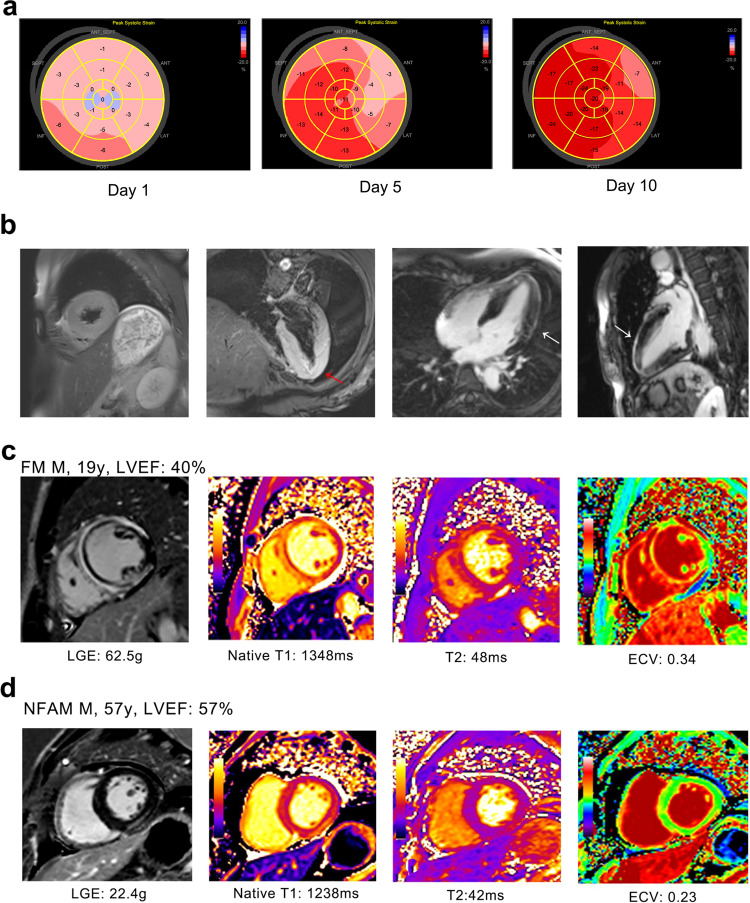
Representative echocardiography and CMR assessment images of clinical FM patients. a regional strain distribution of FM patients on the day of admission, the fifth day and tenth day of hospitalization. Note the regional strain distribution improved following appropriate treatment under the guidance of life support-based comprehensive treatment regimen. b Representative CMR image of FM patients. T2WI showed left ventricular hypertrophy and massive left ventricular wall edema (red arrow) in a 37-year-old FM female patient. The middle section of apex and left ventricular wall showed late gadolinium enhancement (LGE) signal (white arrow), which indicates massive myocardial injuries. c, d Representative CMR image of a FM patient (c) and a non-fulminant myocarditis patient (d). Note the increased diffuse LGE pattern of FM compared to non-FM patient. Longer native T1 and T2 and higher extracellular volume fraction (ECV) were observed in FM patients. Figures 4c and d is from ref. ^104^ with the permission of the *Journal of Magnetic Resonance Imaging*
